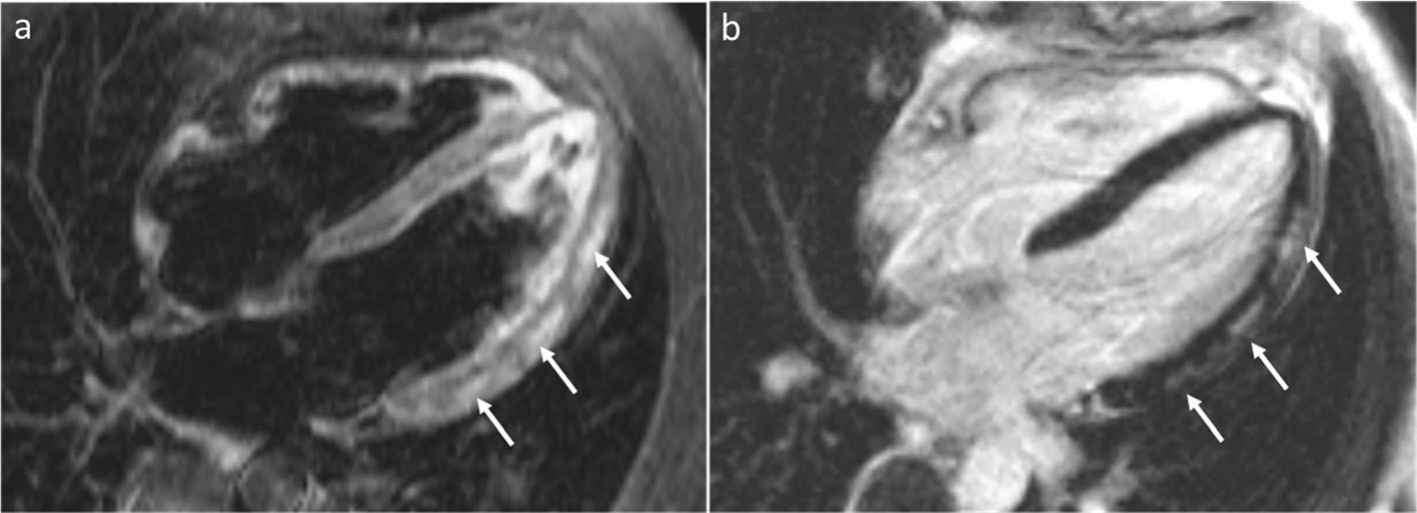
Acute Myocarditis. Four chamber long-axis view T2-weighted image (a) and corresponding late gadolinium enhancement (LGE) image (b). The white arrows indicate patchy epicardial and mid-wall areas of myocardial edema a with corresponding epicardial and mid-wall late enhancement (b)
